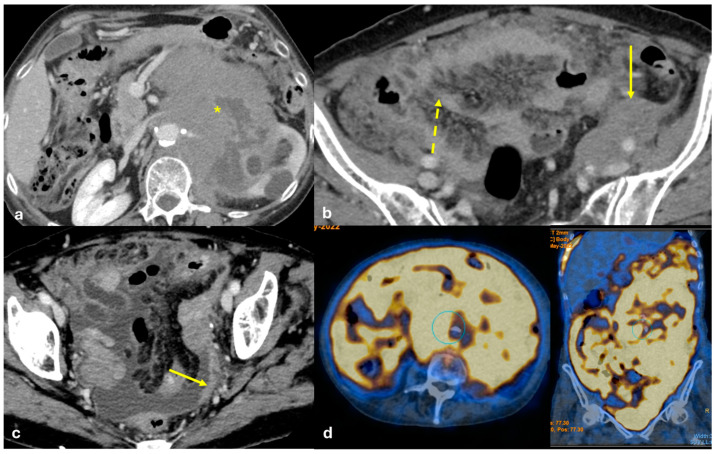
Peritoneal lymphomatosis (large B-cell lymphoma). A middle-aged woman with large B-cell lymphoma involving the left kidney, the pancreas, and the retroperitoneal space ((a), asterisk) with peritoneal lymphomatosis. CECT on the axial plane shows omental caking ((b), dashed arrow), solid tissue along the left iliac vessels ((b), arrow), and peritoneal seeding along the peritoneum which covers the pelvic wall ((c), arrow). (FDG) PET-CT shows diffuse FDG uptake of the lymphoma and peritoneal diffusion sites (d).
